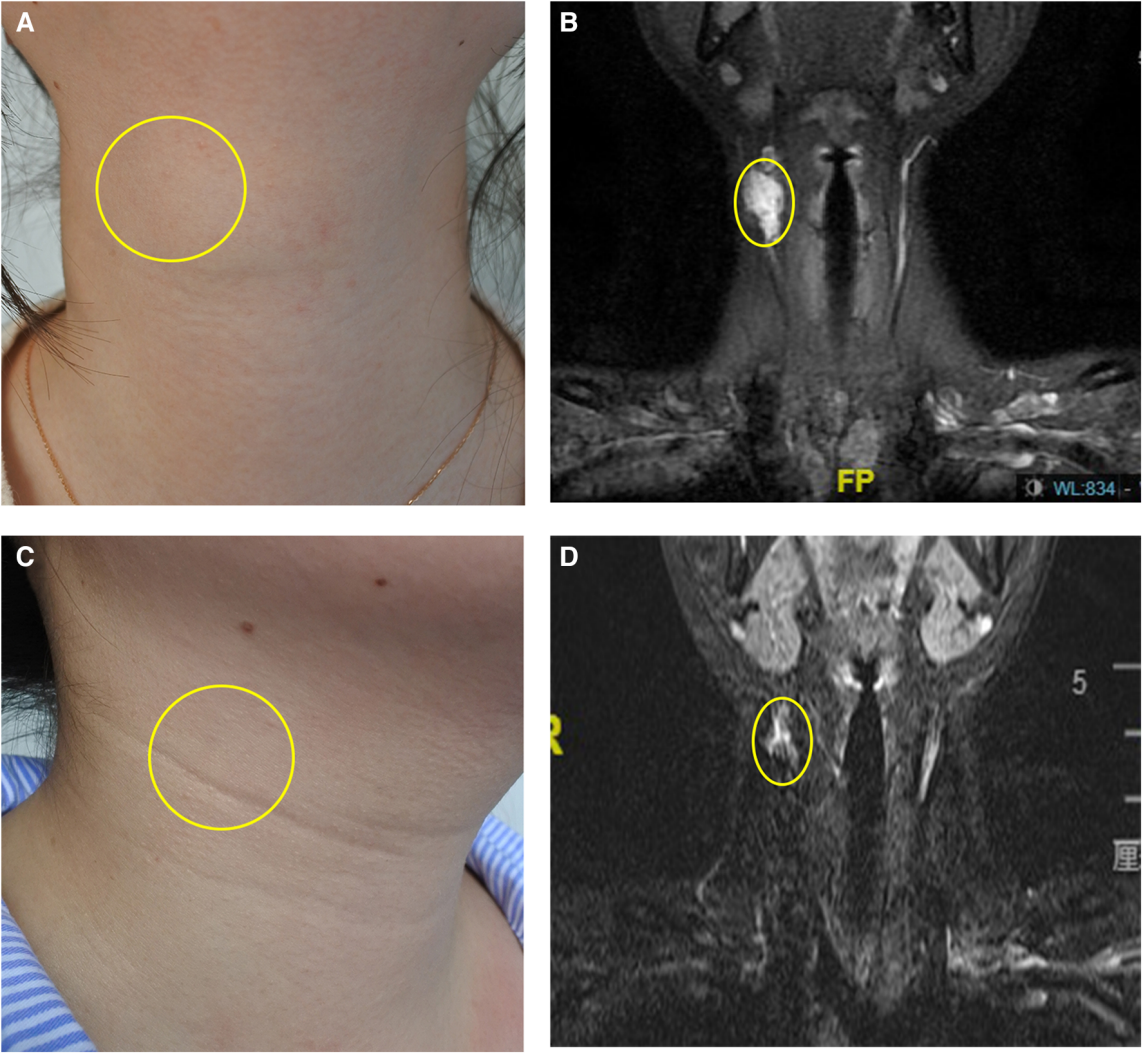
Focal mass type IMVM in the right neck. (A) The appearance before treatment. (B) The MRI before treatment. (C) The appearance after two times of treatment. (D) The MRI after treatment. The yellow ellipse indicated the lesion. The copyrights of the figure were held by corresponding author.
